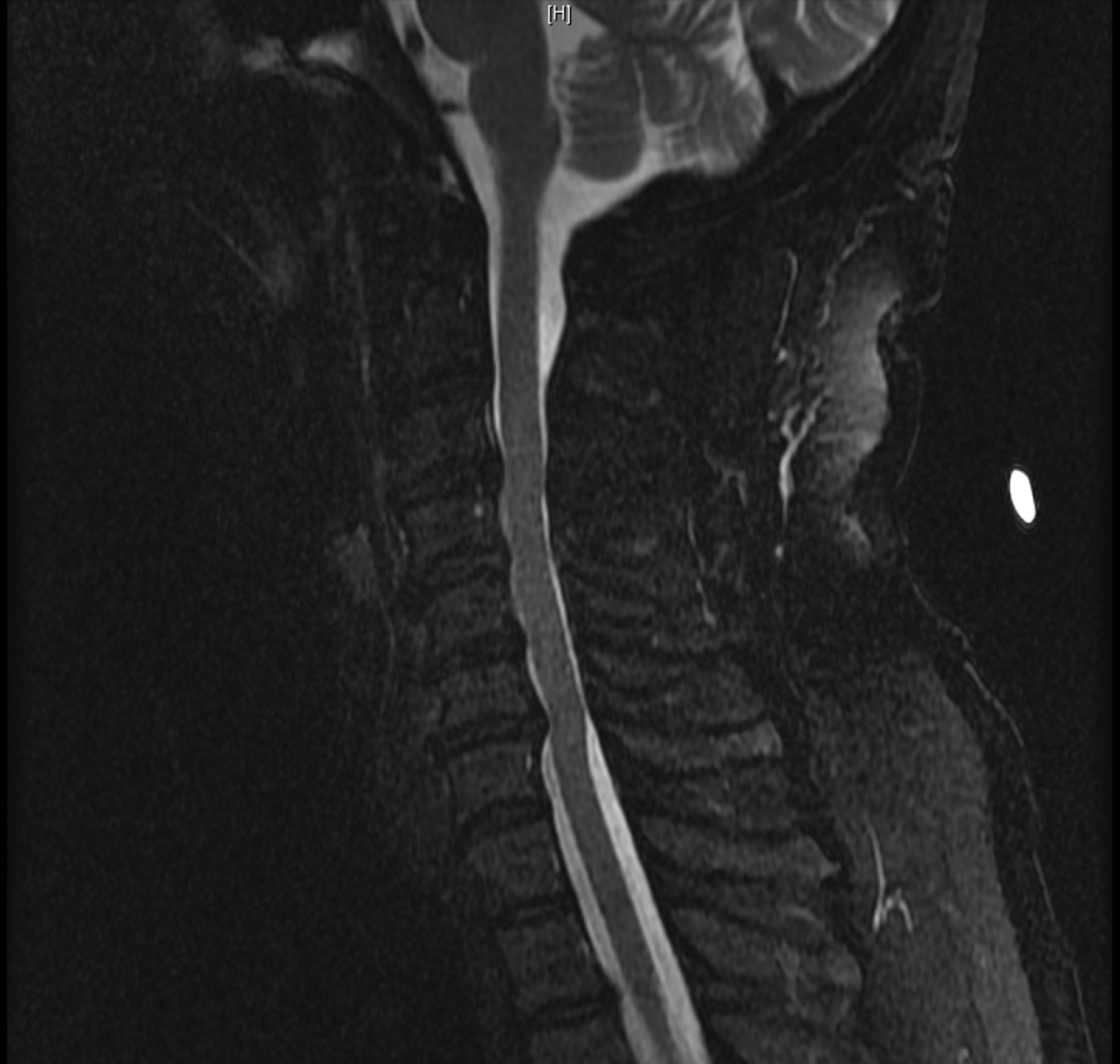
Sagittal T2-weighted MRI showing narrowing of spinal canal at C3-C7.
